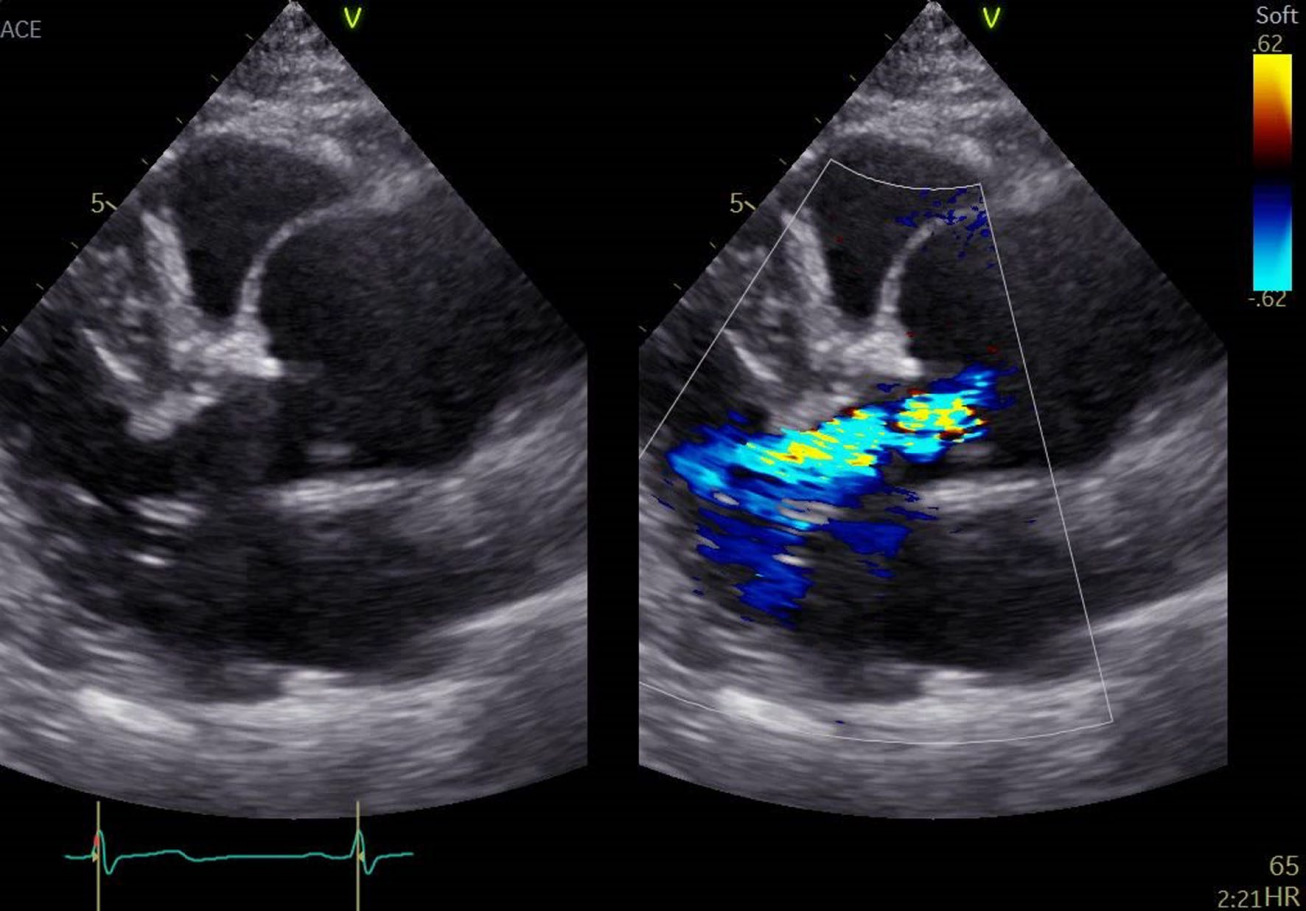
Aortic regurgitation due to annuloaortic ectasia on transthoracic echocardiogram
